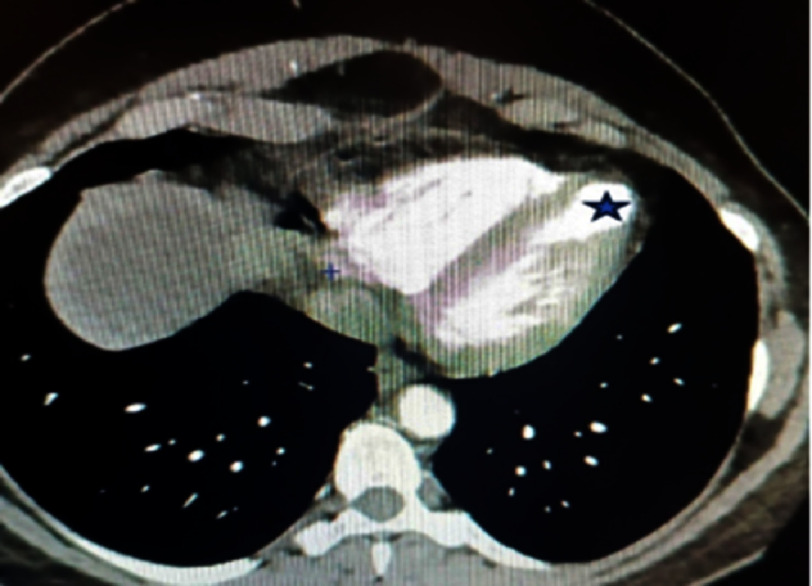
Aspect of left ventricular apical aneurysm (star) in a contrasted chest computed tomography scan.
